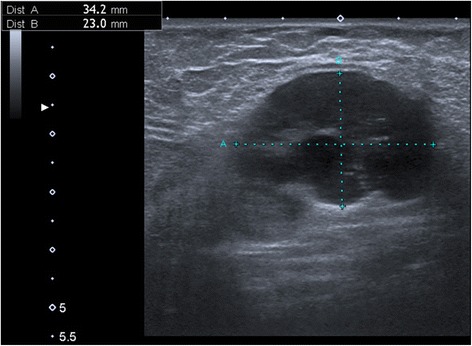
Ultrasound image of enlarged lymph node in the left axilla
